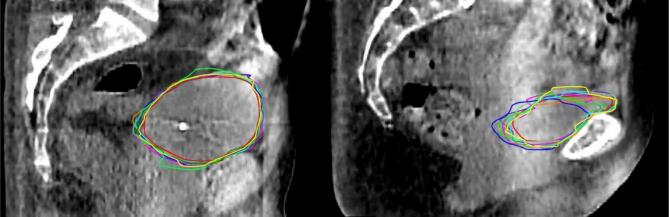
Sagittal view of CBCT with contours of CTV-T_GT_ (yellow), CTV-T_AI_ (red), CTV-T_ADP_ (remaining colours) for patient 1 (left) and 10 (right), with relatively low and high inter-observer delineation variation, respectively. CI_gen_ was 0.88 for patient 1 and 0.71 for patient 10. Both patients have given written consent to use of images and treatment plans. (For interpretation of the references to colour in this figure legend, the reader is referred to the web version of this article.)
